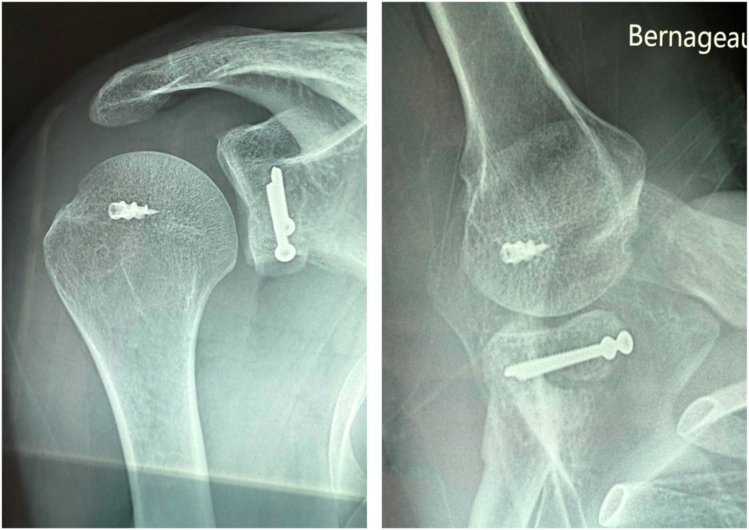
Post-operative radiographs of an anterior glenoid bone block combined with Hill-Sachs remplissage.
